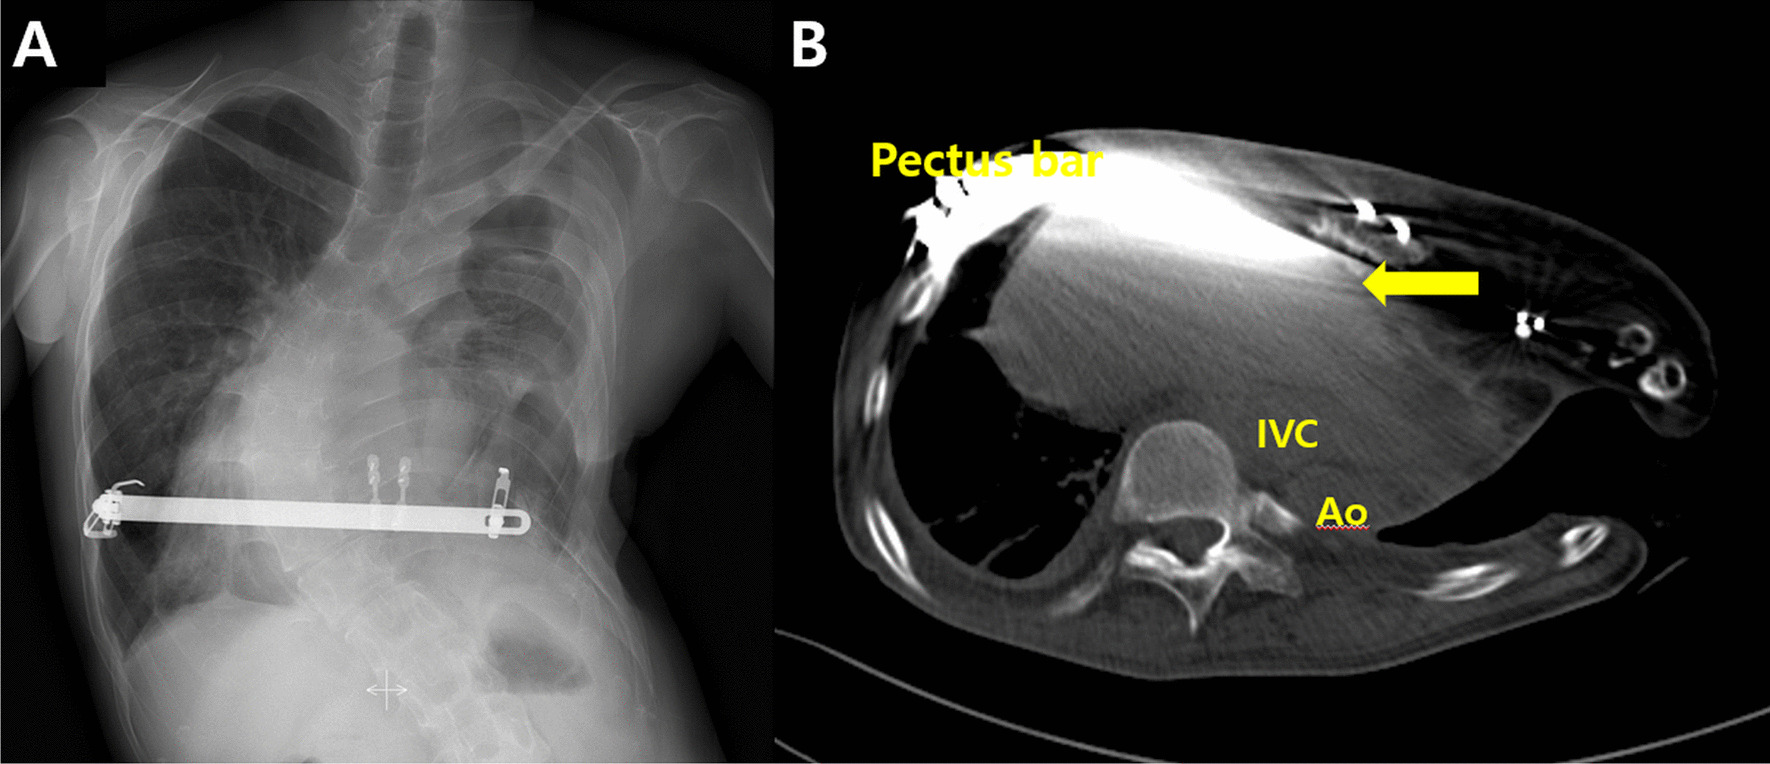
Images after the modified Ravitch procedure. A A simple chest x-ray shows the modified Ravitch procedure with a pectus bar. The tracheostomy had been removed. B Chest computerized-tomography six months after the Ravitch procedure shows successful relief of compression on the heart and inferior vena cava by the depressed chest wall (IVC: inferior vena cava, Ao: descending aorta, arrow: most depressed point)
